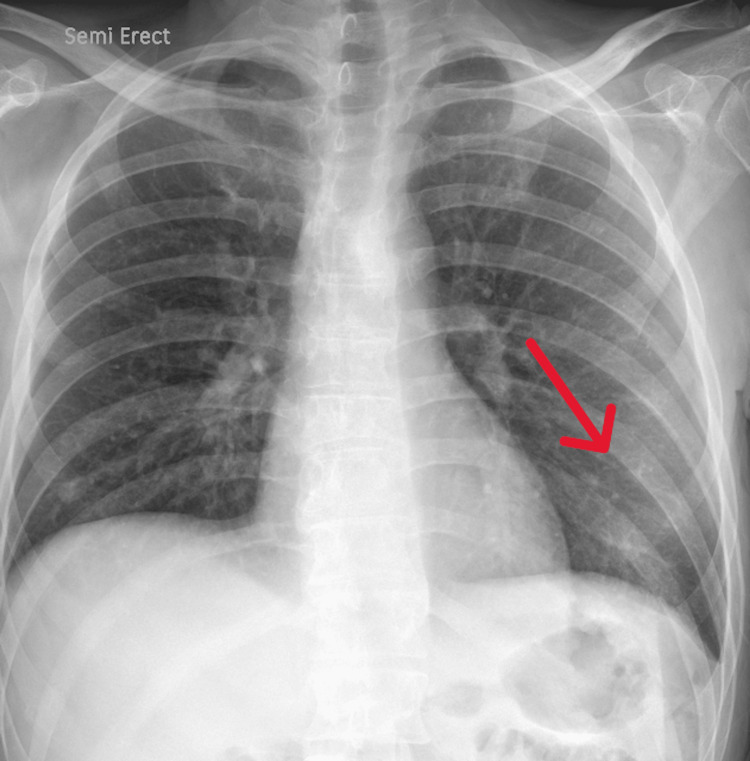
X-ray of the chest showing left lobe infiltrates (red arrow) from previous infection
